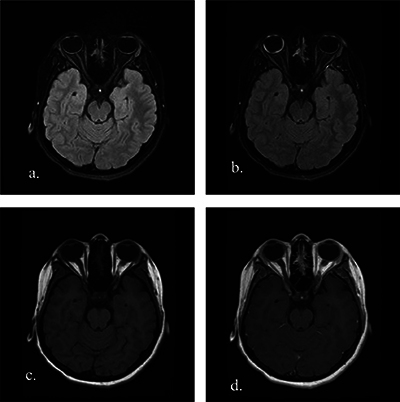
Bilateral panuveitis in a 13-year-old girl with Behcet’s disease. Axial fat-suppressed FLAIR non-enhanced (a) and contrast-enhanced (b) images reveal uveal tract thickening with marked contrast enhancement in the right eye and mild contrast enhancement in the left eye. The increased vitreous signal in the right eye is also noted. Axial non-enhanced (c) and contrast-enhanced (d) T1WI show no definite uveal tract enhancement in both eyes.
